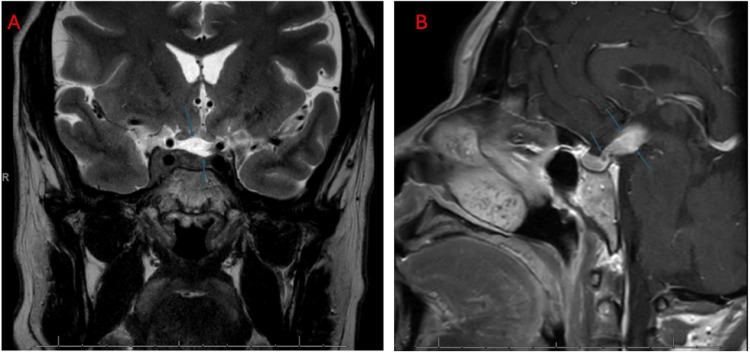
MRI of the brain with and without contrast: coronal (A) and sagittal (B) images demonstrating marked interval enlargement in the suprasellar mass (blue arrows) measuring approximately 3.0×2.6 cm MRI: magnetic resonance imaging
